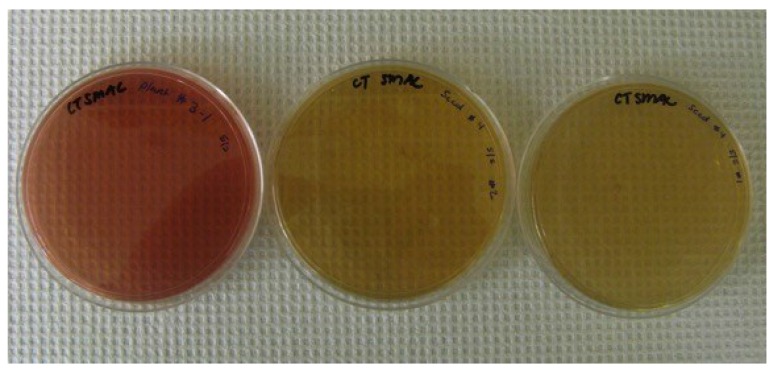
CT-SMAC plates from *E. coli* O157:H7-GFP samples recovered from tomatoes that had been seed contaminated with the bacterium (two plates on the right) and were positive for GFP following PCR. *E. coli* O157:H7 does not ferment sorbitol and appears as white colonies on CT-SMAC plates. As a negative control, cultures were plated that were not positive for GFP. The plate on the left is representative of the plates obtained with colonies all appearing red in color (can ferment sorbitol) indicating that they are not *E. coli* O157:H7.
